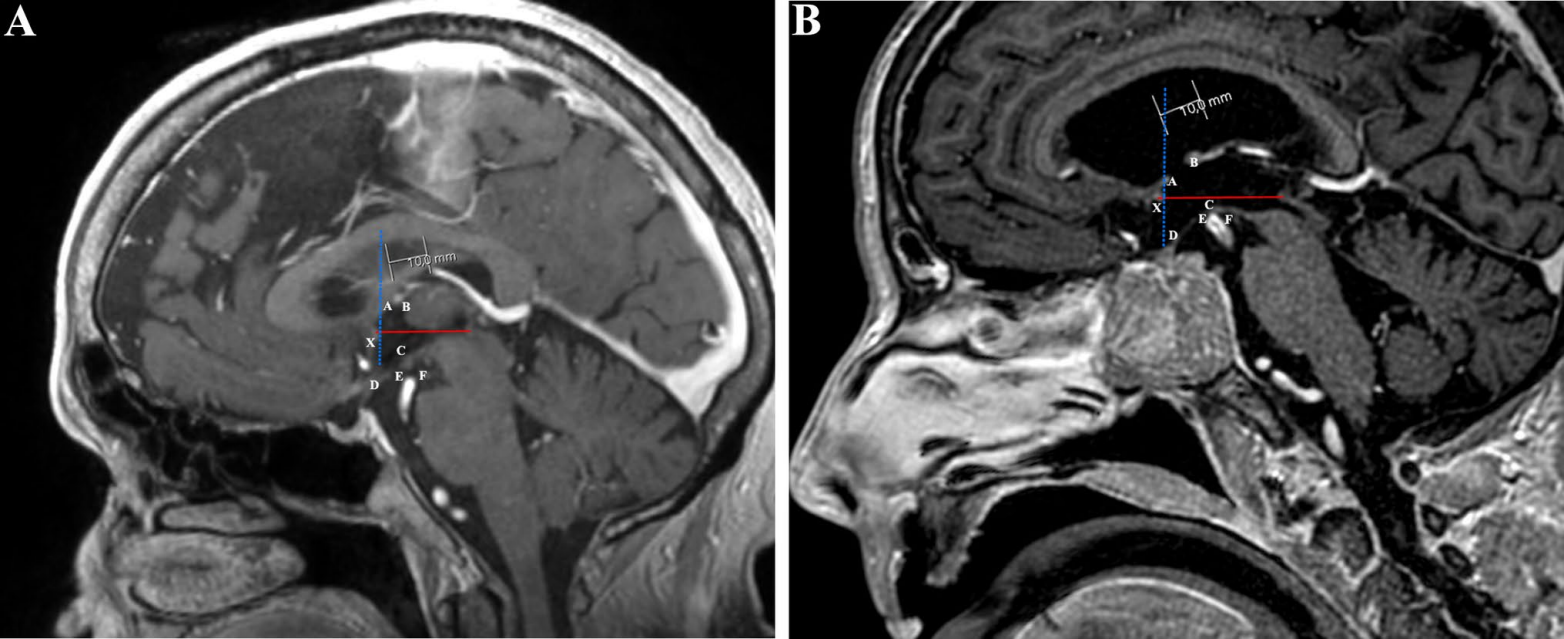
A Brain MRI. 3D T1-weighted post-contrast, midsagittal plane from normal subjects. The same anatomic landmarks were used as reference points. The anterior commissure-posterior commissure line was drawn (red line) and chosen as *Y*-axis. The *Z*-axis (blue dotted line) was drawn passing through the posterior and superior portions of the anterior commissure. The intersection of both axes defined the stereotactic reference point (X). Six additional anatomic points (A–F) were used in the characterization of the anatomy of the third ventricle. B Brain MRI. 3D T1-weighted post-contrast, midsagital plane from subjects with hydrocephalus. The same anatomical landmarks were used as reference points. A—Midline projection of the anterior border of the interventricular foramen; B—Midline projection of the posterior border of the interventricular foramen; C—Mammillary bodies anterosuperior portion; D—Most inferior point of the infundibular recess; E—Most superior point of the basilar artery; F—Midline projection of the emergence of the oculomotor nerve. Scale bar 10 mm (colour figure online)
